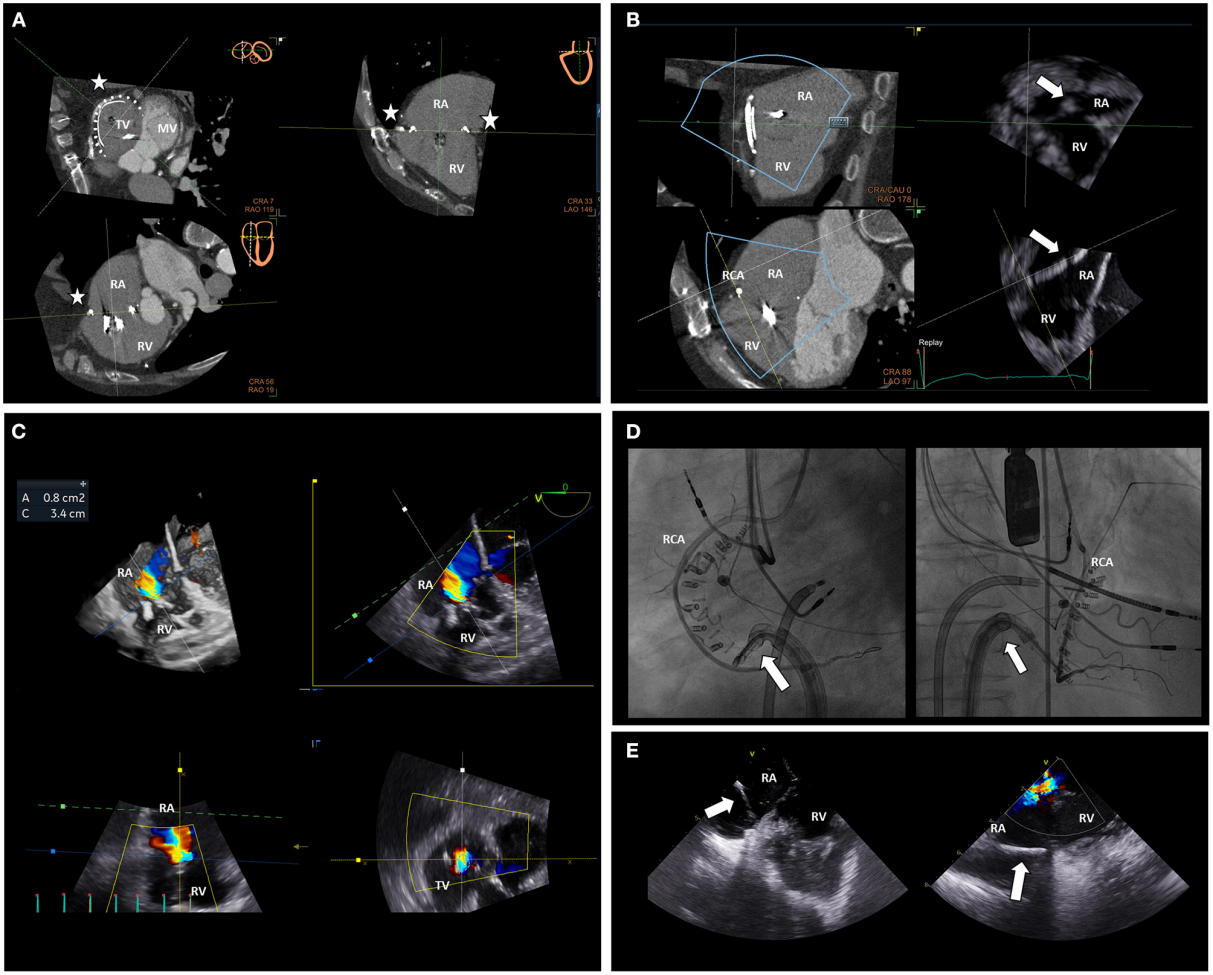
Multimodality imaging for intra-procedural guidance of a Cardioband procedure on the tricuspid valve. MDCT data displaying the predicted position of the anchors of the Cardioband device (stars) can be aligned with real-time echocardiographic data (A) during the procedure. The MDCT-echocardiography fusion imaging permits a better alignment of the delivery system (arrow) to place the anchors in the predicted position on the TA (B) avoiding potential complications such as RCA compression or damage. TR severity and TA dimensions can be assessed during the procedure with multi-planar reconstruction echocardiography (C) to monitor the result. Orthogonal fluoroscopic views can be used to locate the delivery system (arrows), the anchors of the Cardioband device and their relative position to the RCA (D). Moreover, intracardiac echocardiography can be very helpful to align the delivery system (arrows) to the TA and aim for an optimal position of the anchors (E), especially in the case of challenging transesophageal echocardiographic views or difficult anatomy. MV, mitral valve; RA, right atrium; RCA, right coronary artery; RV, right ventricle; TV, tricuspid valve.
